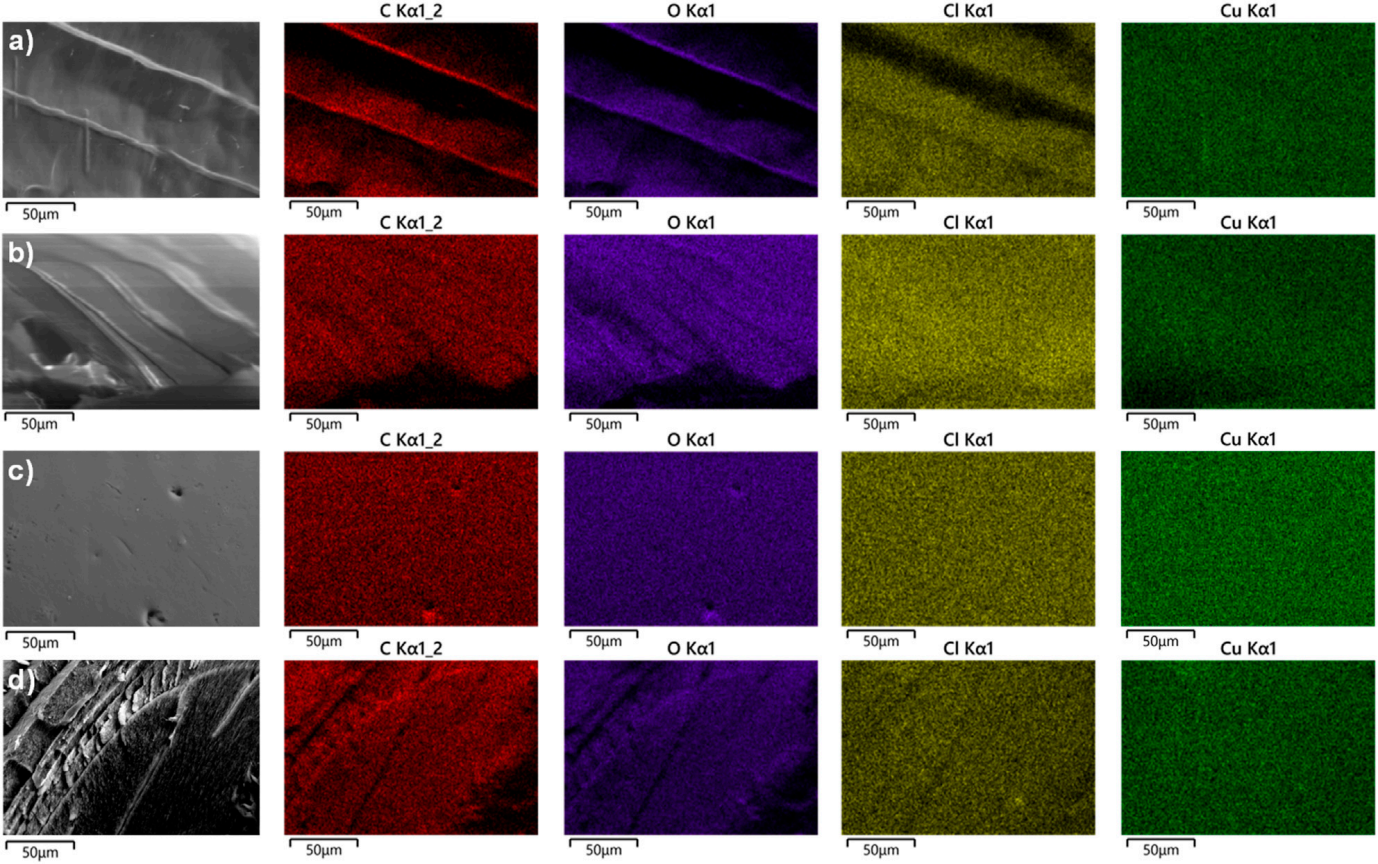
Energy-dispersive X-ray mapping images of the (a) Cu(II)-AXG surface; (b) Cu(II)-AXG cross-section; (c) Cu(II)-AAG surface; and (d) Cu(II)-AAG cross-section.
